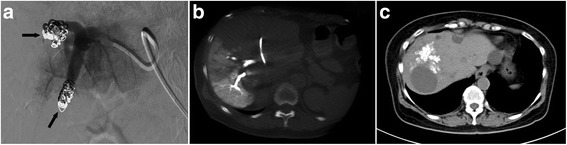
Effect of terminal branch portal vein embolization. a Post-embolization venogram with coils visible in the anterior and posterior right portal vein (*black arrows*). b CT scan showing embolized terminal branches of the right portal vein. c CT scan showing lipiodol deposition
